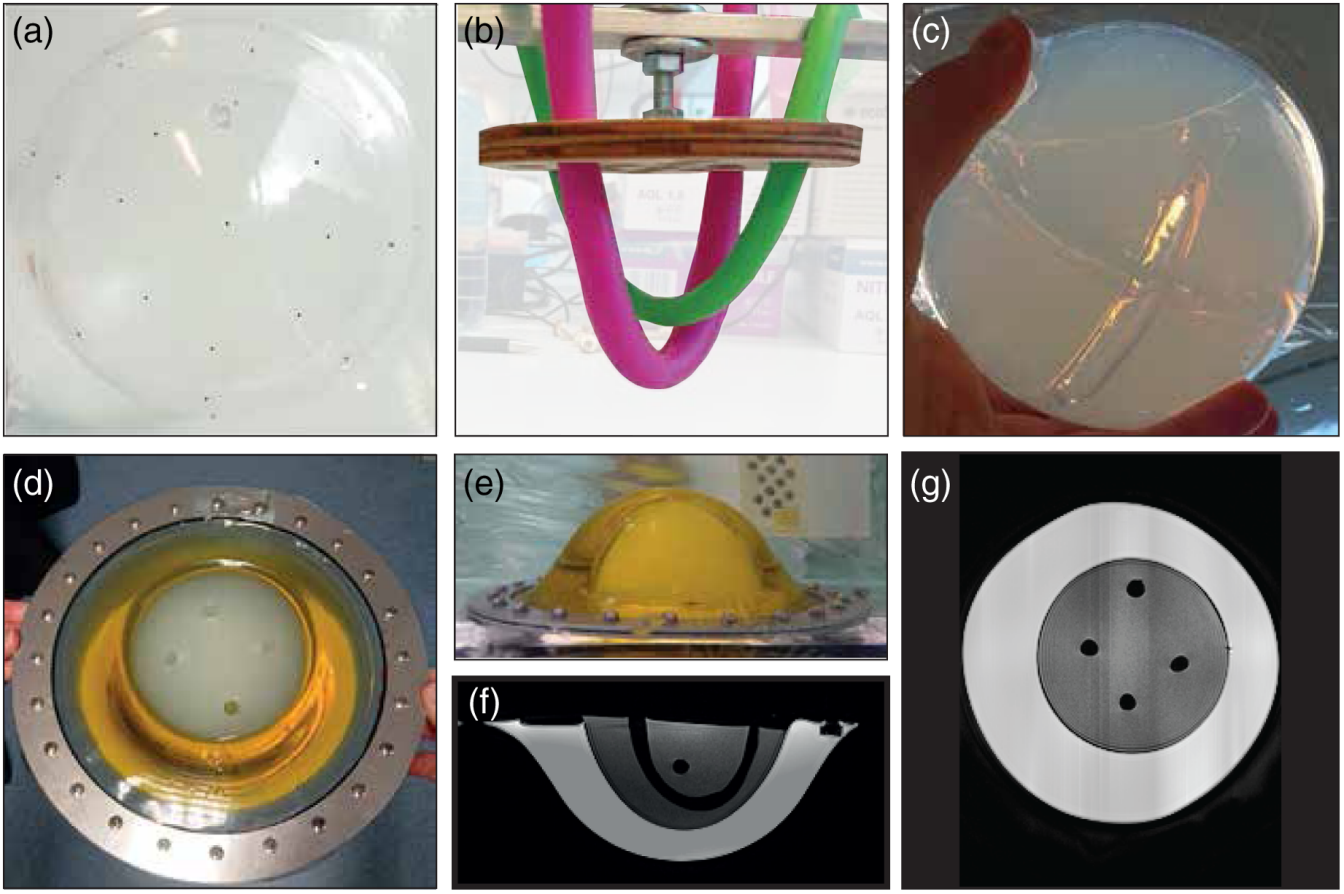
Pictures taken during and after the production of the SOS object. (a) Subresolution beads glued to the ellipsoid cup. (b) Modeling balloons filled with water as placeholders for the channels in the PAG. (c) The polyacryl amide gel volume after draining and removing the balloons. (d), (e) Top view and side view of the assembled SOS object, showing the olive oil and PAG compartments. The channel openings in the PAG are also visible. (f), (g) Cross sections of an MRI scan made of the object, showing the object’s build-up.
